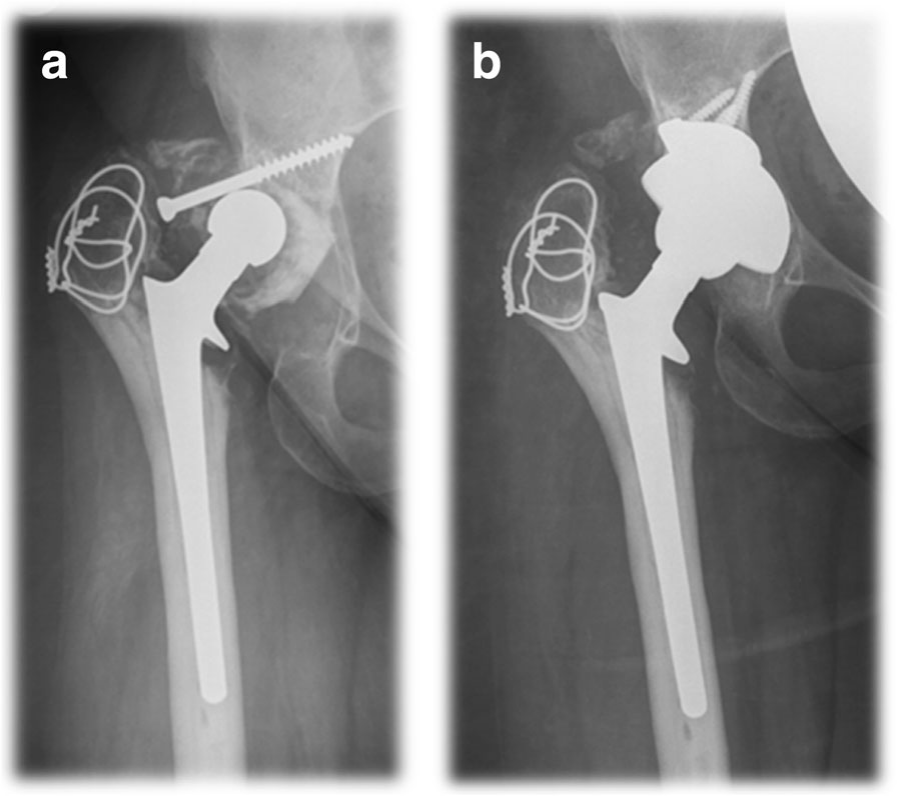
a Preoperative anteroposterior radiograph in a 34-year-old patient who underwent total hip arthroplasty THA for severe dysplastic osteoarthritis and presented with aseptic loosening of the polyethylene cup. b Anteroposterior radiograph of the same patient 5 years postoperatively, with a trabecular metal wedge and shell
